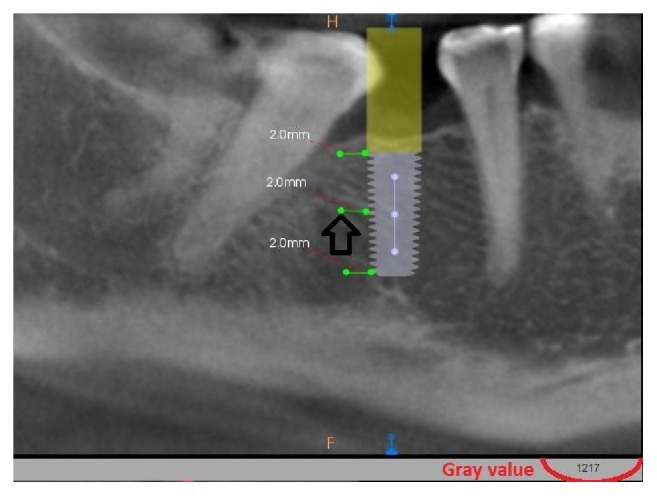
Calculation of grey value in CBCT. Dark arrow indicates the value of grayscale measured in the area of arrowhead (results highlighted in red color).
